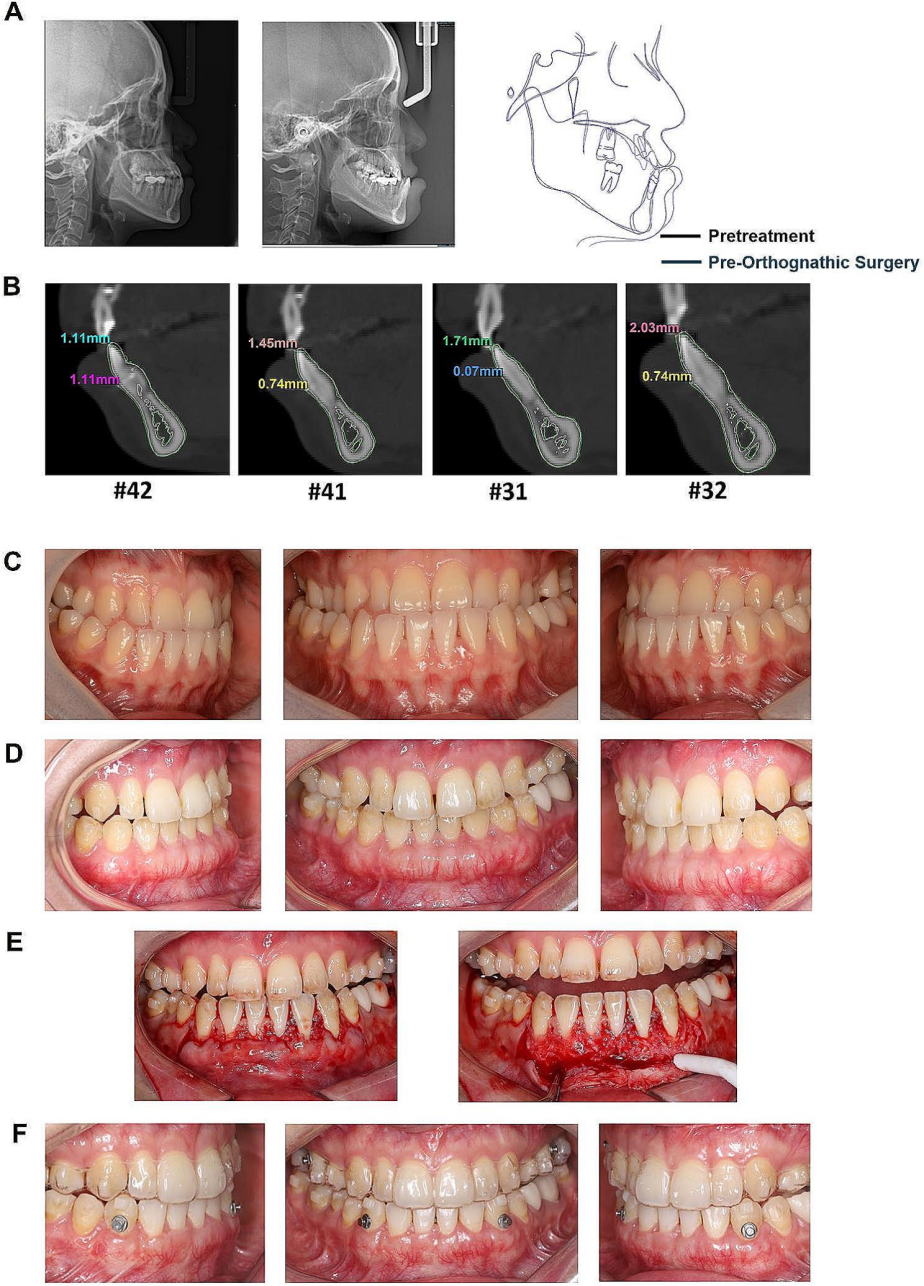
Cephalometric radiographs, CBCT and clinical photographs of case 4. A The comparison of the cephalometric radiographs of the baseline and the end of pre orthognathic surgical orthodontic treatment. B The superimposition images of the #31#32#41#42 CBCT data at baseline and at the end of pre orthognathic surgical orthodontic treatment. C The appearance of the anterior mandibular teeth with thin periodontal phenotype at baseline. D The photographs at the end of orthognathic surgical treatment. E A second surgical procedure aimed at restoring the gingival contour to its desired shape were conducted. Biopsies were obtained from beneath the soft tissue flap and from the exact surface of the alveolar bone. F The photographs at three months after the surgery aimed at restoring the gingival contour
